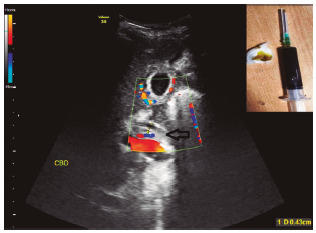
Duplex ultrasonography showing small-caliber CBD lacking adequate vascularity
